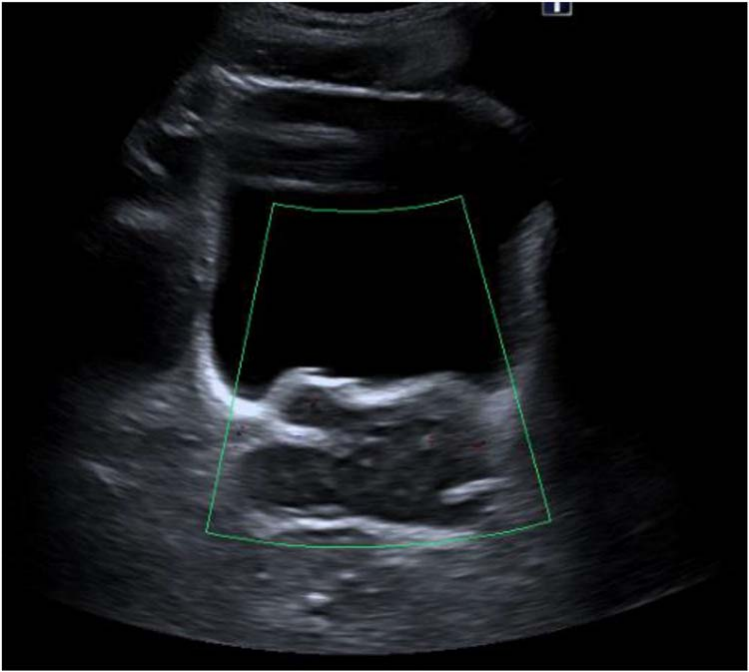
Renal ultrasound showing enlarged prostate indenting into the bladder.
